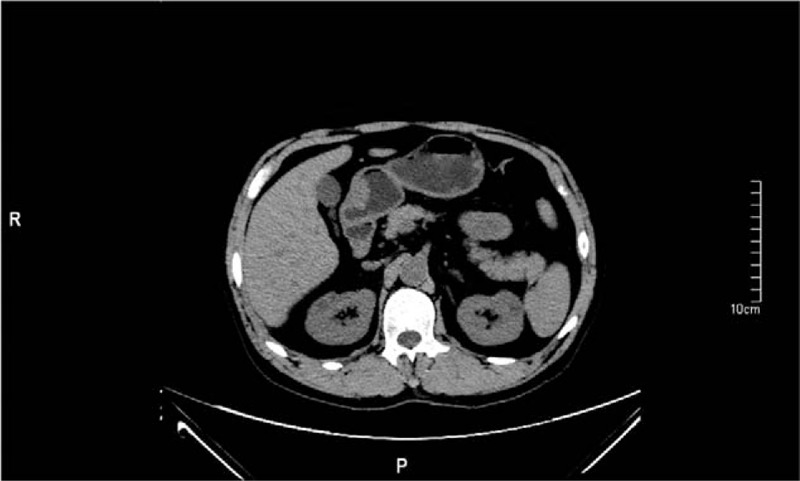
Iconography findings. Abdominal CT scan showed that there is a thickening in the front wall of the gastric antrum. CT = computed tomography.
